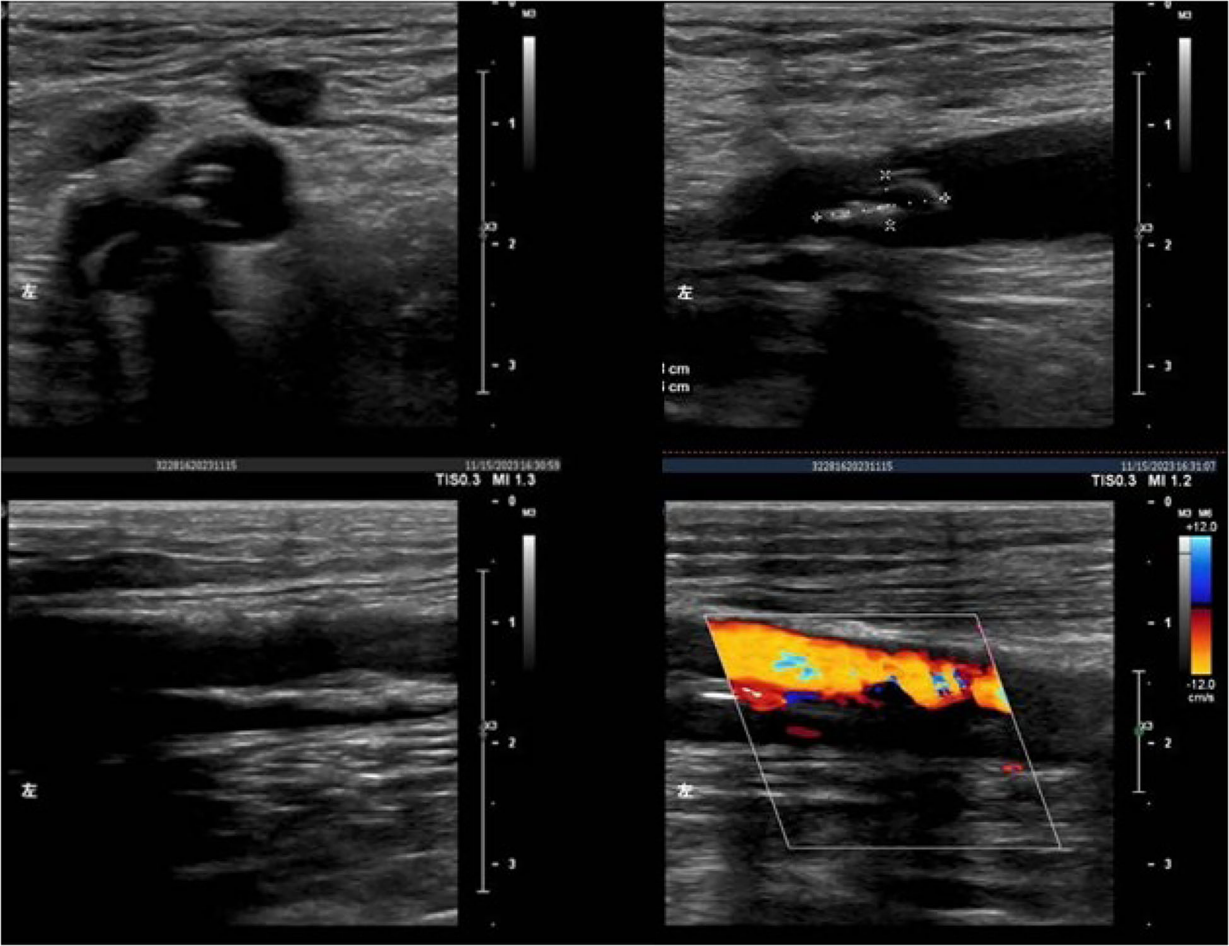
Ultrasound examination of the left lower extremity artery. The course of the left common femoral artery, the superficial femoral artery, the deep femoral artery, and the popliteal artery was normal. The entire vessel wall was not smooth. Multiple high-echo and low-echo plaques were observed in the left common femoral artery and superficial femoral arteries. One high-echo plaque was approximately 1.08 x 0.43 cm in size. Low-echo fillings were present in the left anterior tibial, posterior tibial arteries, and peroneal arteries.
